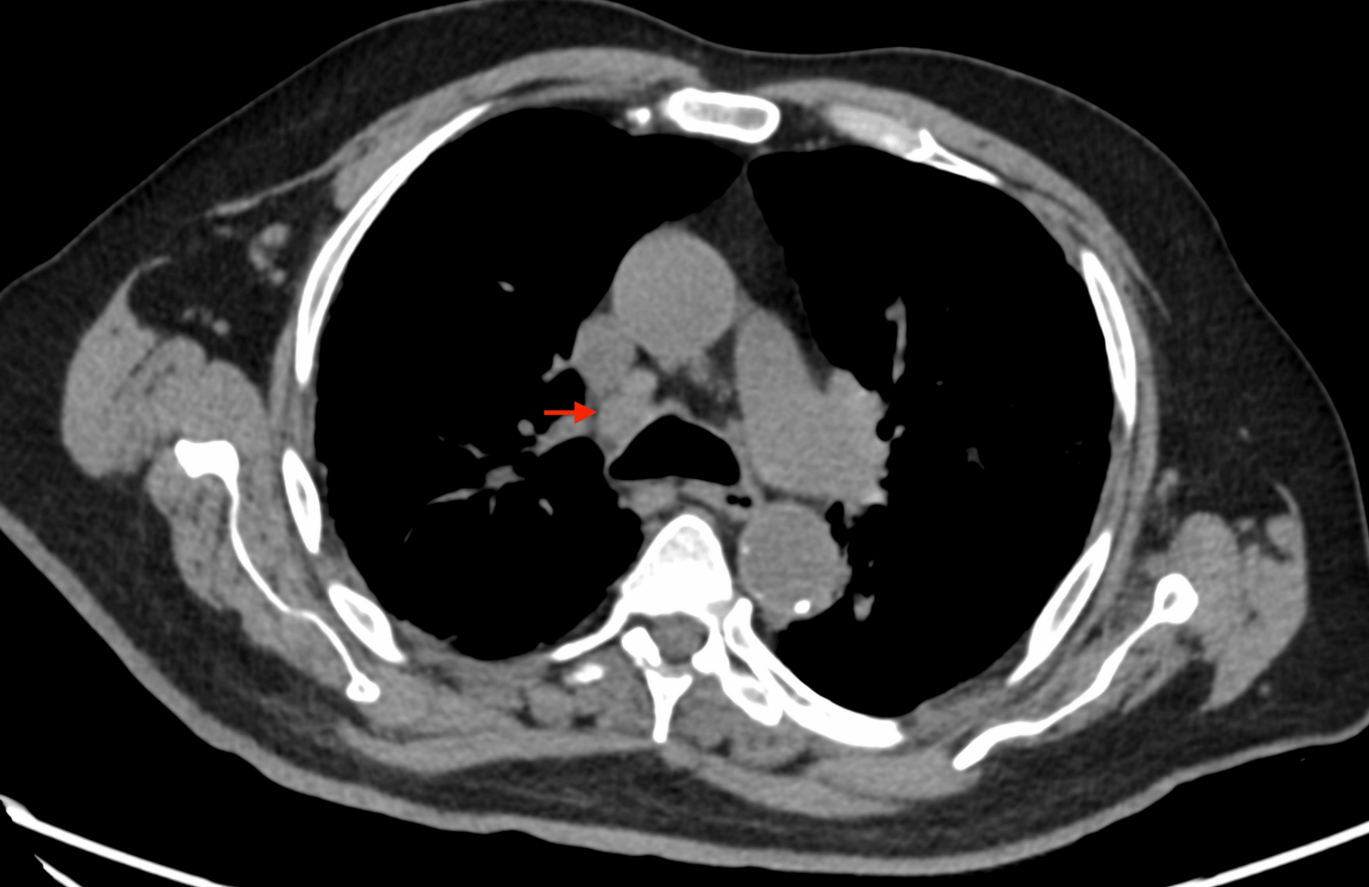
Computed tomography (CT) scan of the chest showing enlarged mediastinal lymph nodes The red arrow represents an enlarged pretracheal lymph node.
